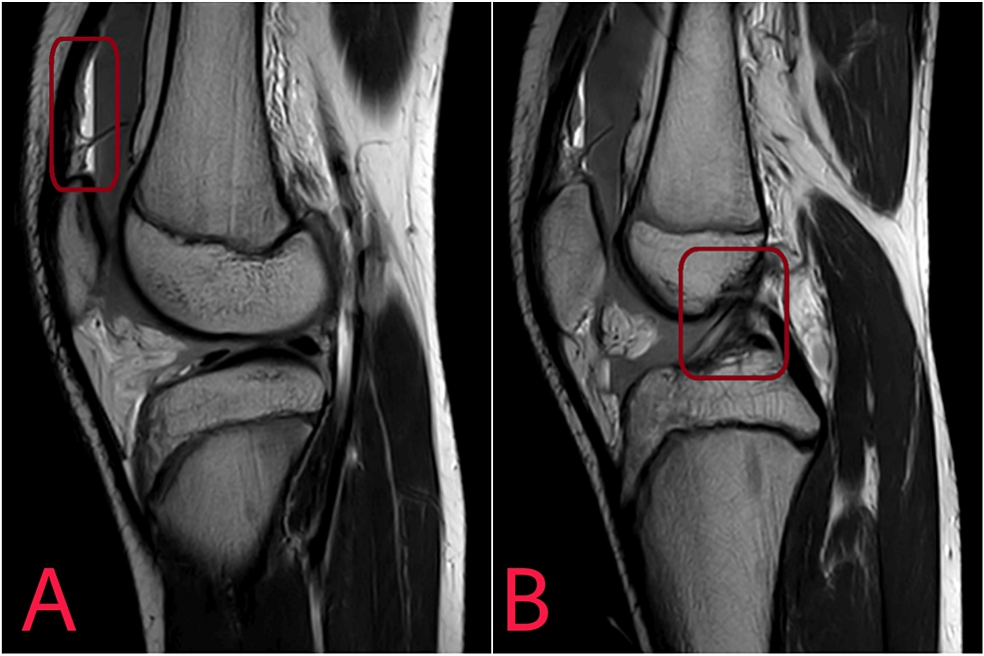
An MRI showing suprapatellar lipohemarthrosis (A) and anterior cruciate ligament tear (B).
